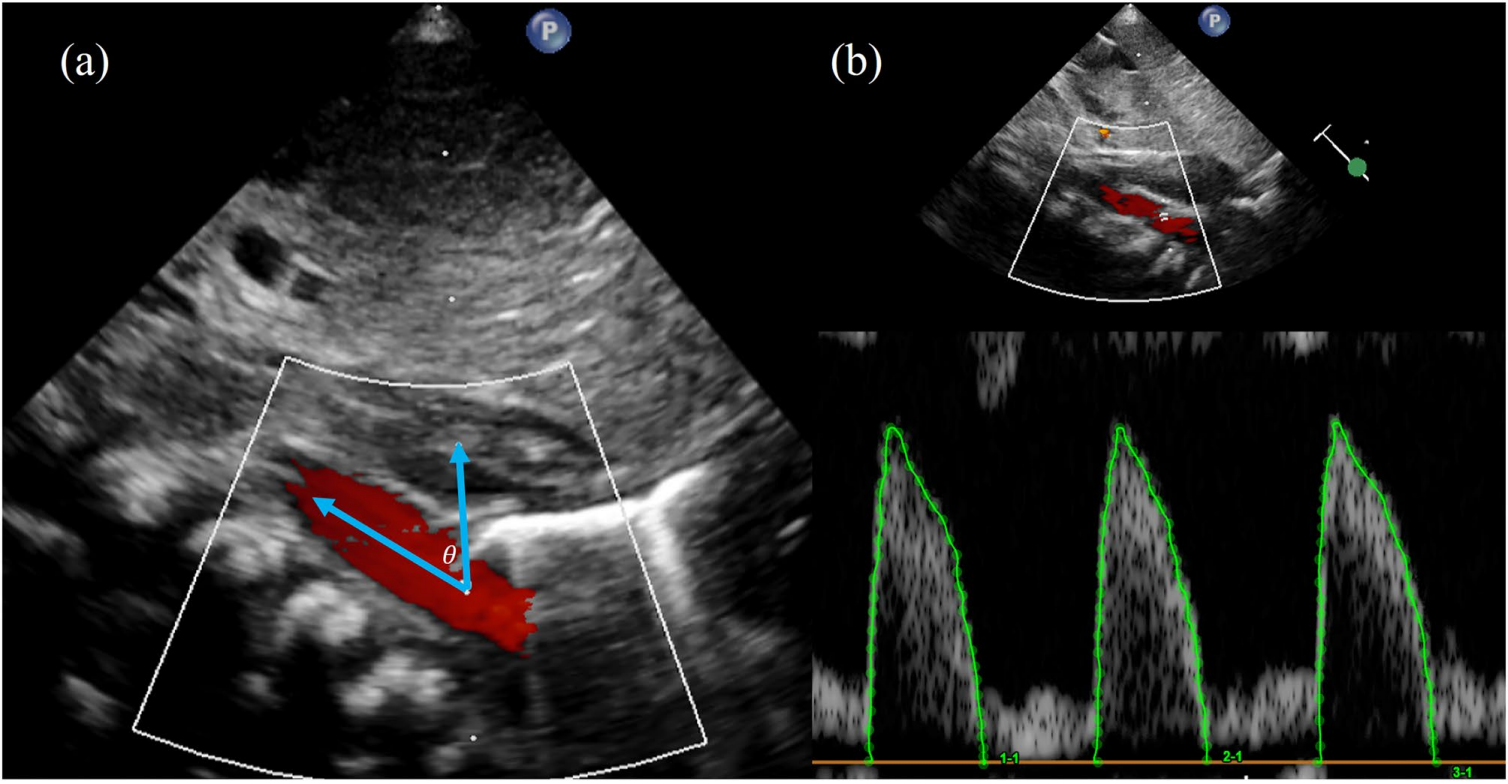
Echocardiographic images for obtaining descending aorta blood flow. (a) A subcostal sagittal view showing descending aorta (Ao) blood flow at the level of diaphragm (D). Two arrows indicate the directions of blood flow (left) and ultrasound beam (right) with an angle of *θ*. (b) The VTI was obtained by tracing pulsed-wave Doppler image and divided by cos *θ* for angle correction between blood flow and ultrasound beam. Abbreviations *VTI* Velocity time integral.
